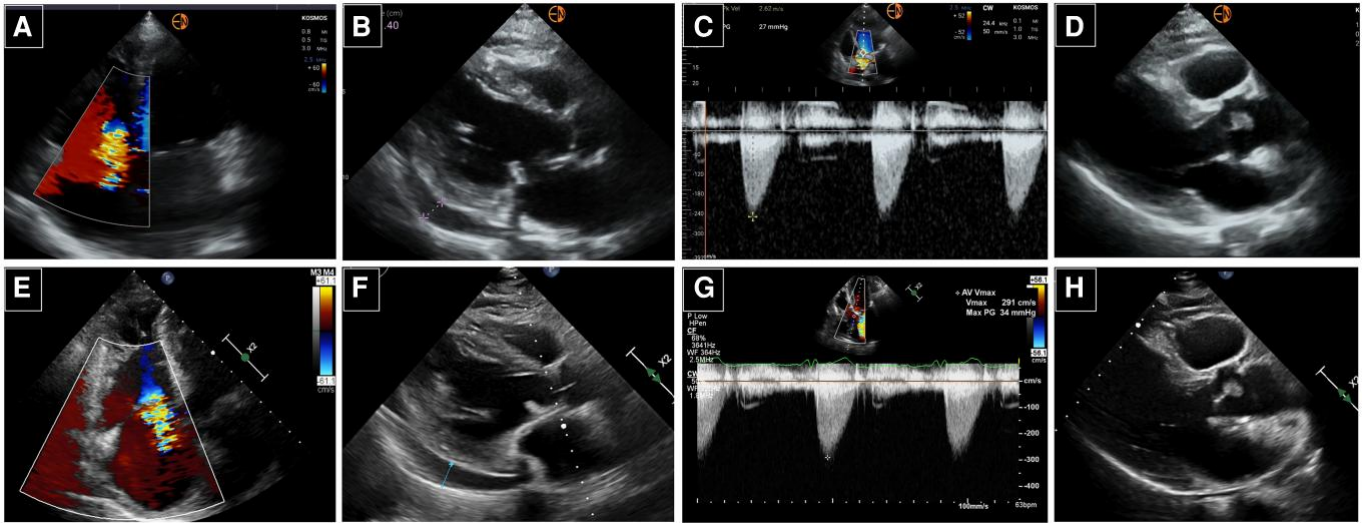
Apical four-chamber view obtained using HUD (*A*) and SE (*E*) in a patient with severe tricuspid regurgitation. Parasternal long-axis view obtained using HUD (*B*) and SE (*F*) in a patient with moderate pericardial effusion. Aortic peak jet velocity measured with CW Doppler on five-chamber view using HUD (*C*) and SE (*G*) in a patient with mild aortic stenosis. Parasternal long-axis view obtained using HUD (*D*) and SE (*H*) in a patient with papillary fibroelastoma.
